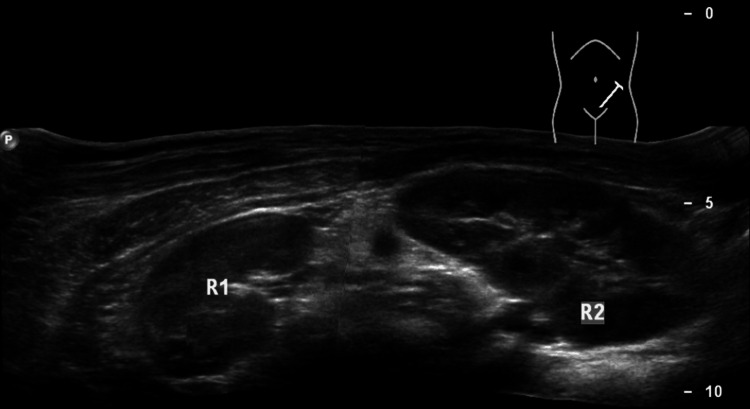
En bloc kidney allograft on ultrasound three years after transplantation
